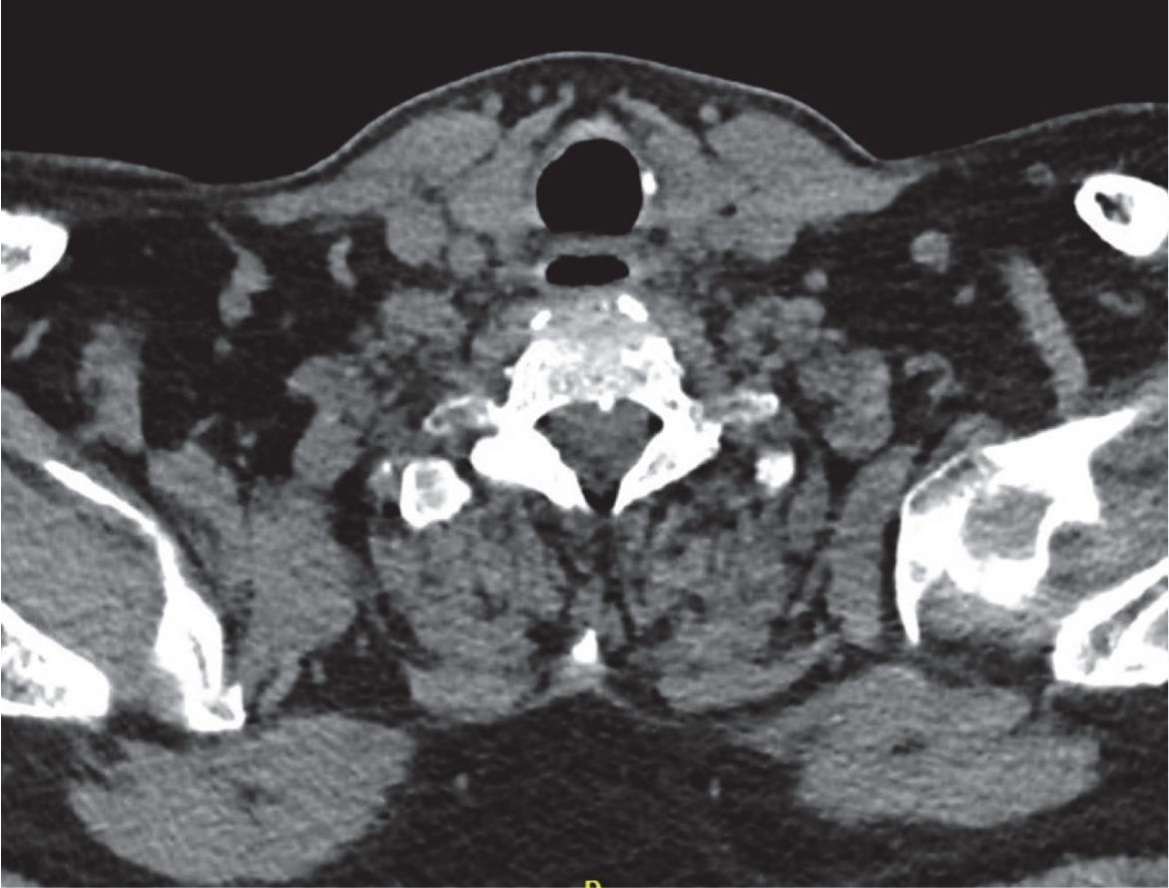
Axial image of neck CT with oral contrast (1 mm slice thickness), showing resolution of thyroid pneumatosis and no contrast extravasation.
